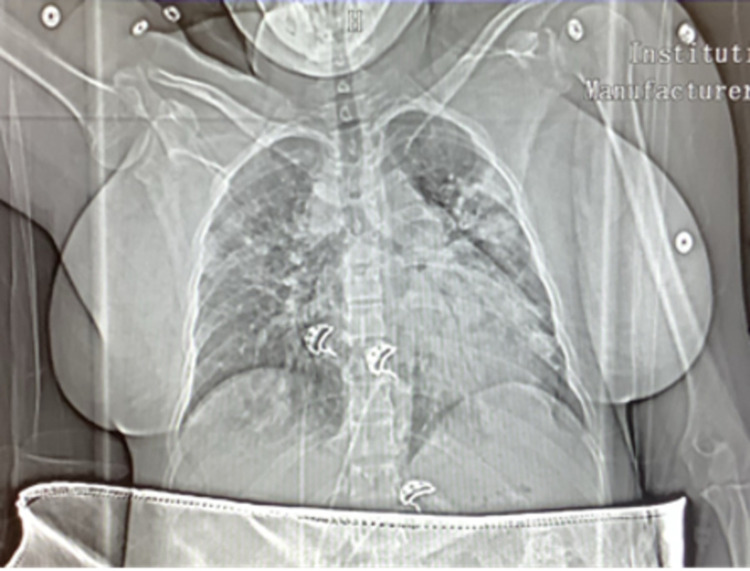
CT angiogram chest showing no evidence of pulmonary emboli and extensive patchy multifocal pneumonia consistent with COVID-19 pneumonia.
